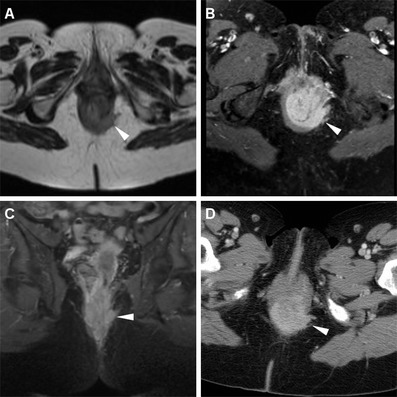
A 62-year-old female with biopsy-proven SCAC. Axial T2-weighted (a), post-contrast fat-suppressed axial (b) and coronal (c) T1-weighted images, and corresponding enhanced image from body CT (d) show a 5.5-cm long (T3) enhancing tumour with infiltration of the left ischioanal fatty space (*arrowheads*)
